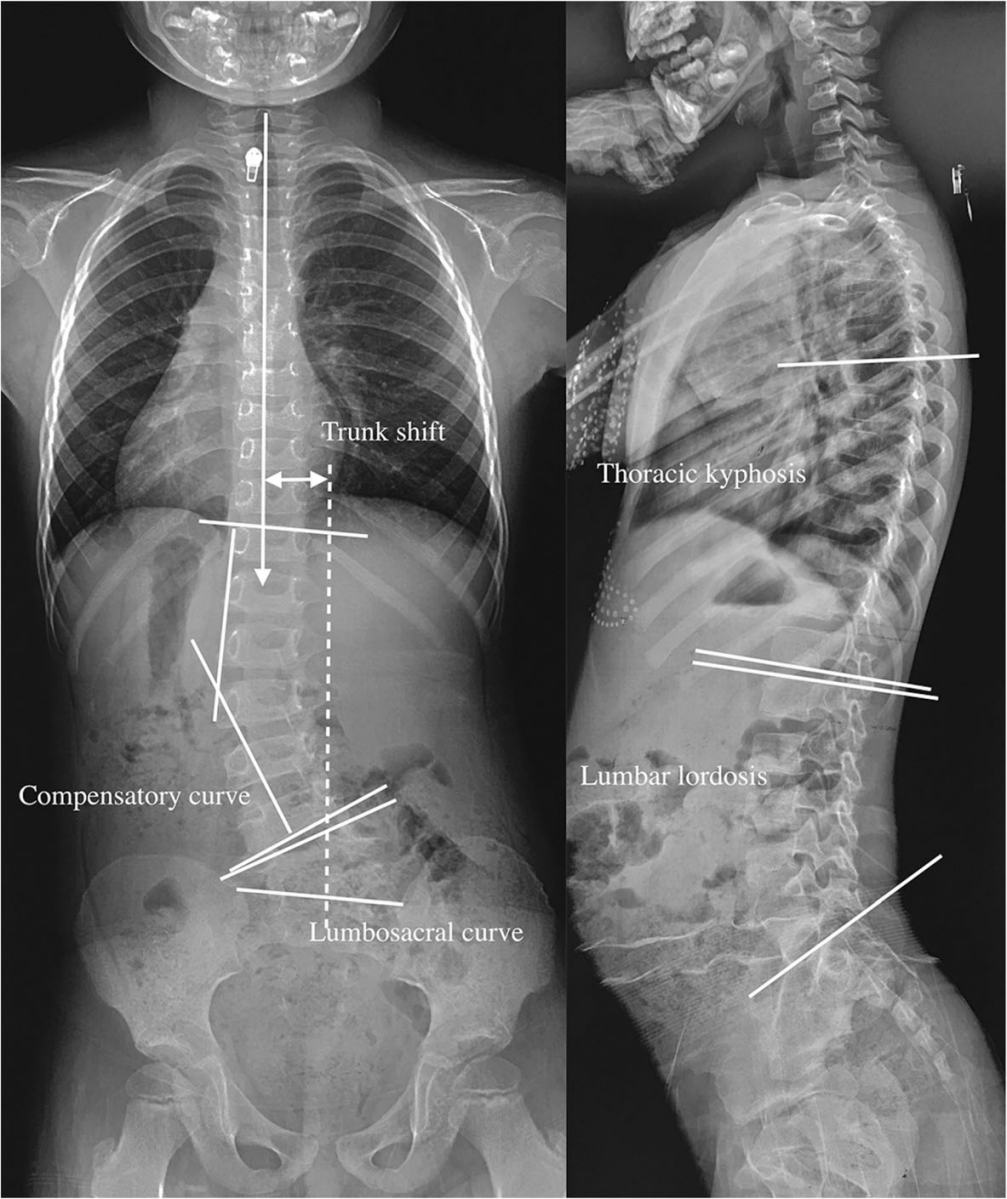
The coronal and sagittal parameters measured on standing whole spine X-rays
